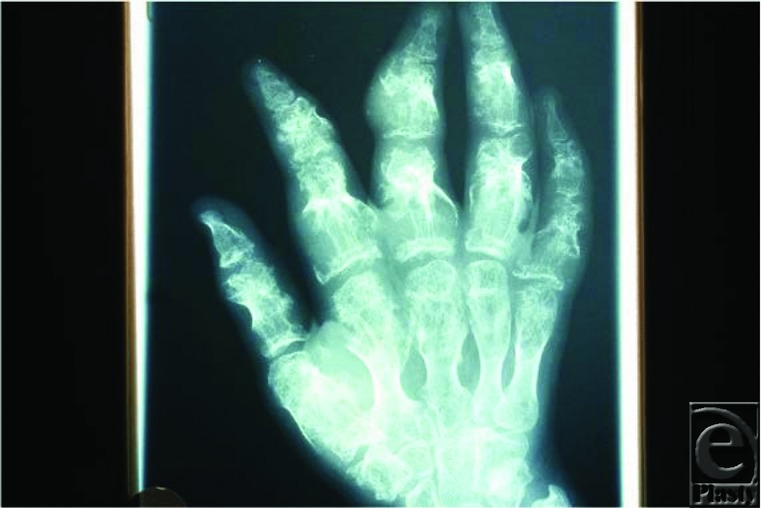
Radiograph demonstrating multiple enchondromas and bony deformation.
